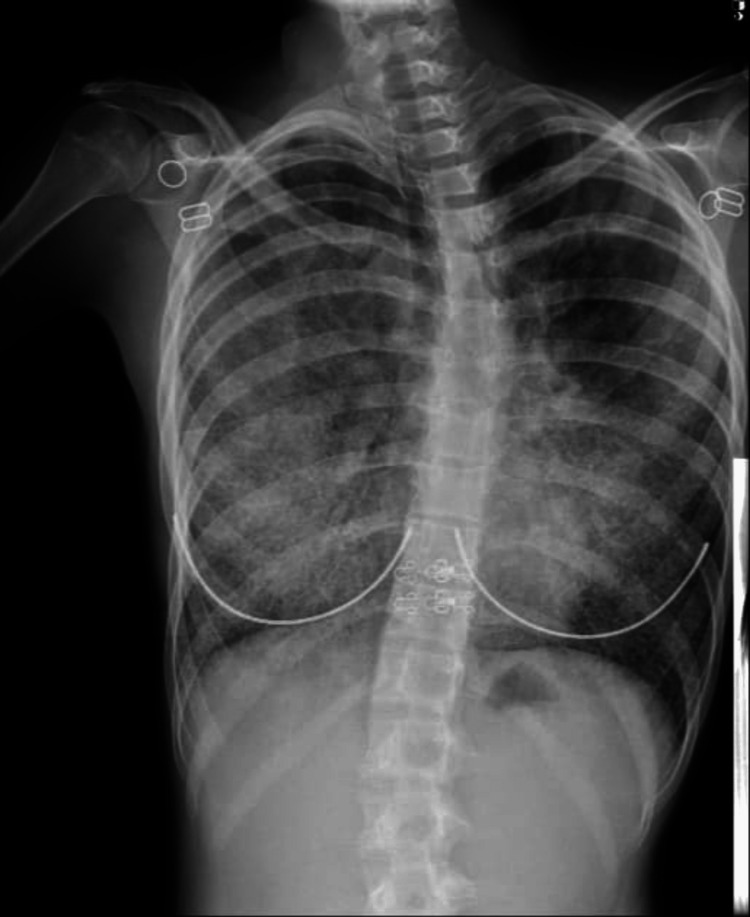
Chest x-ray at presentation with bilateral infiltrates.
